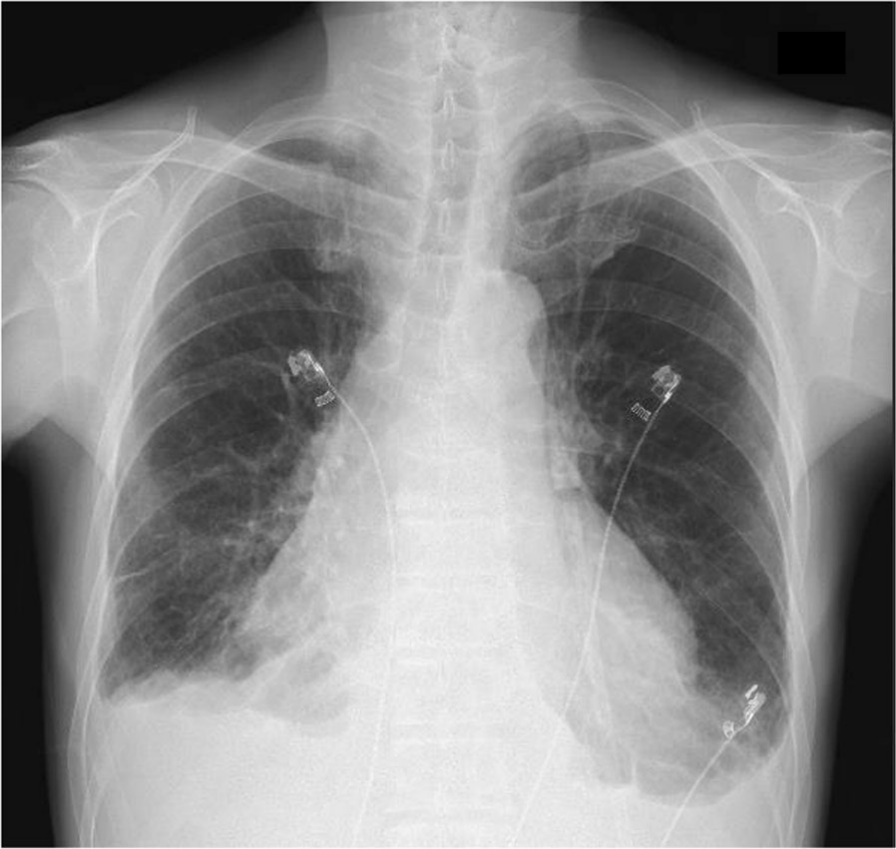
Chest X-ray showed a high cardiothoracic ratio of 63%
